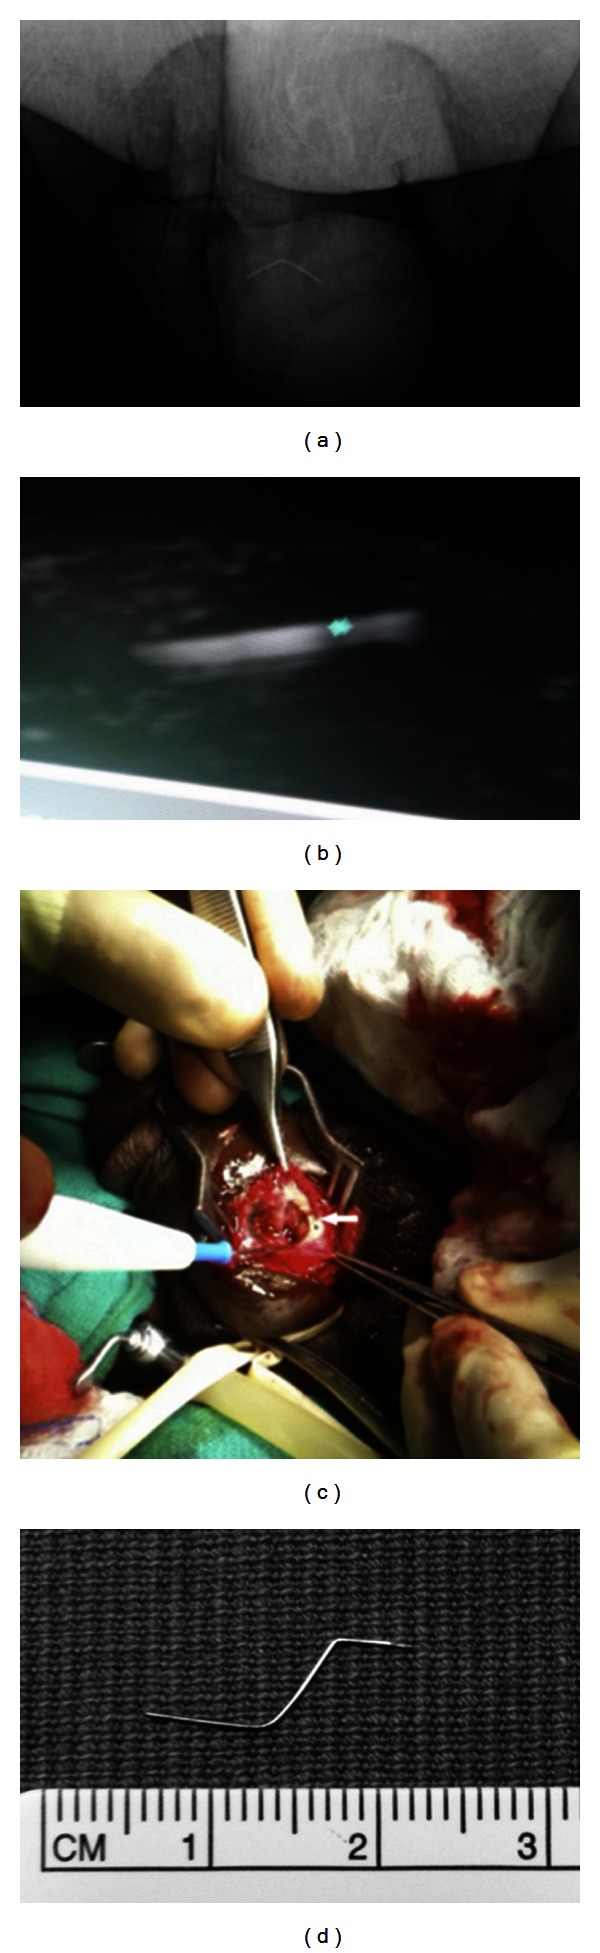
(a) Use of digital radiography to enlarge and increase brightness of the pelvic X-ray to better demonstrate presence of the retained needle. (b) Image of broken intracavernosal needle deep within penile tissue, via portable high-frequency ultrasound. (c) Intraoperative photograph of needle embedded within penile tissue; white arrow shows needle location. (d) The broken needle after extraction from the patient's penis.
